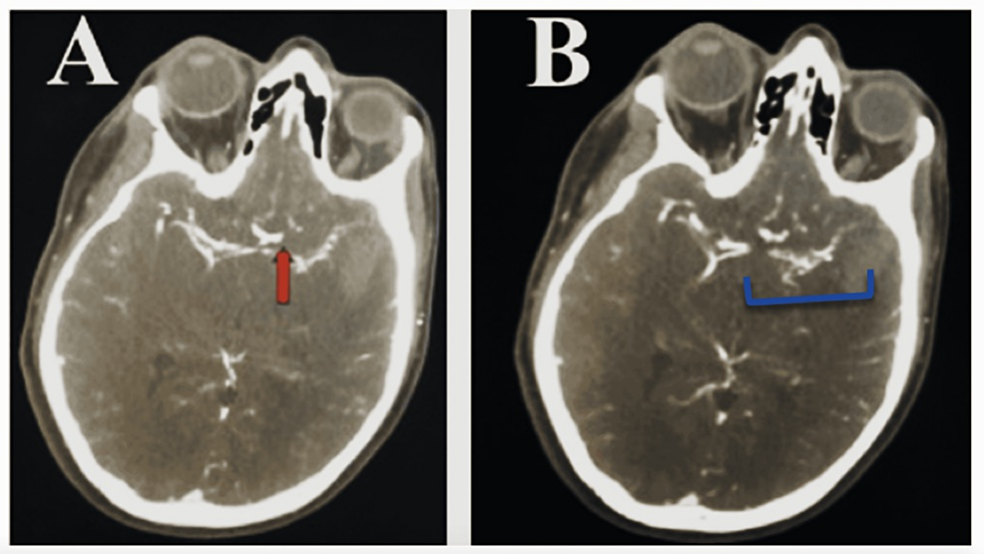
CTA of head and neck showing stenosis and abnormal vascular collaterals. (A) There is an abnormal appearance of the supraclinoid left internal carotid artery at the bifurcation. The arrow points to an area of irregular stenosis along the entirety of the pre-trifurcation of the left middle cerebral artery. (B) The blue bracket encloses the area where there are multiple small vessels arising from this segment of the middle cerebral artery suggesting MMD. CTA: computerized tomographic angiography.
